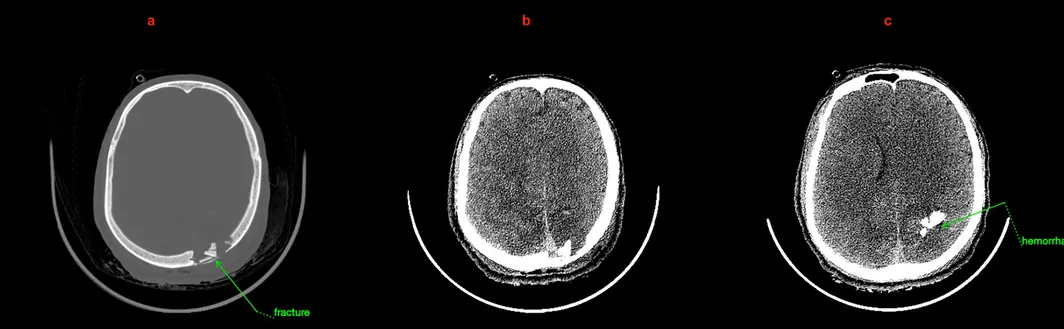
Axial brain CT images in bone (a) and brain (b & c) windows demonstrate a left posterior parietal comminuted and depressed calvarial fracture (green arrow in a) with multiple bone fragments lodged in the underlying brain parenchyma and intraparenchymal contusional hemorrhage and surrounding edema (green arrow in c).
